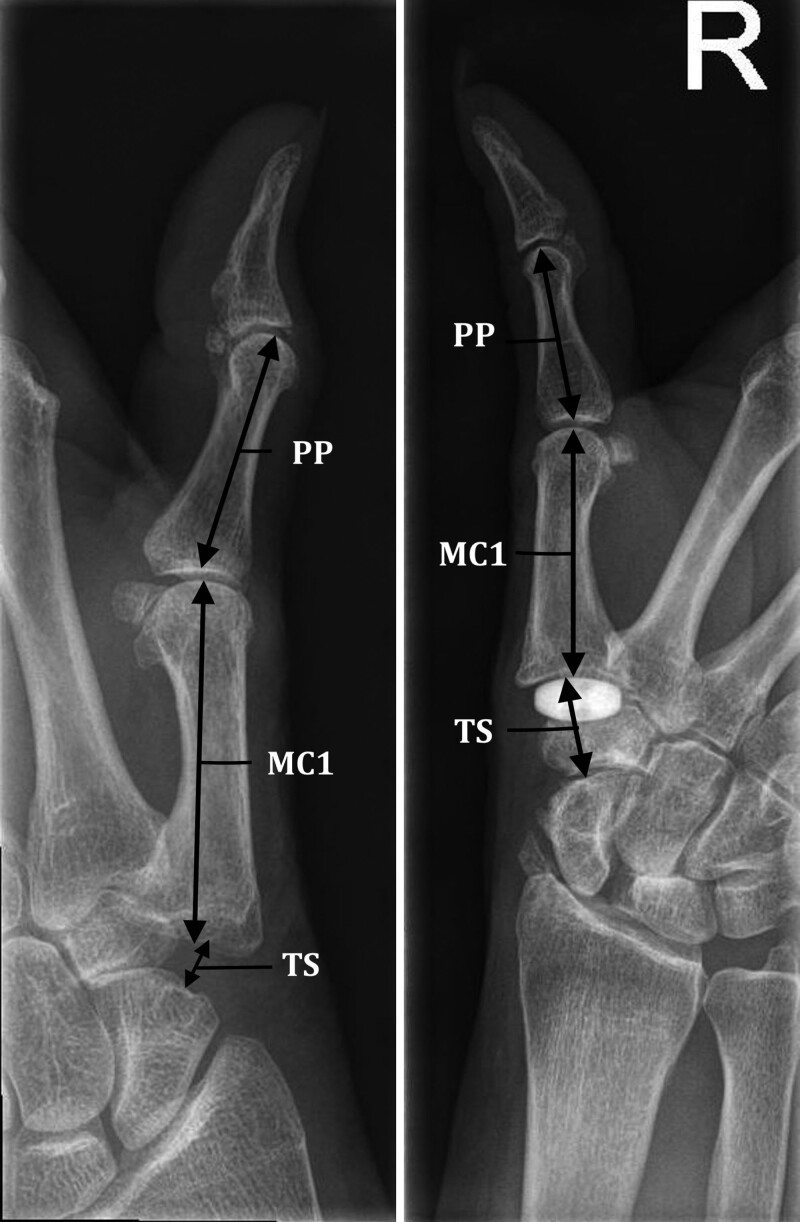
Example of measurement of thumb length on postoperative X-ray. (*Left*) After LRTI. (*Right*) After PDI.
